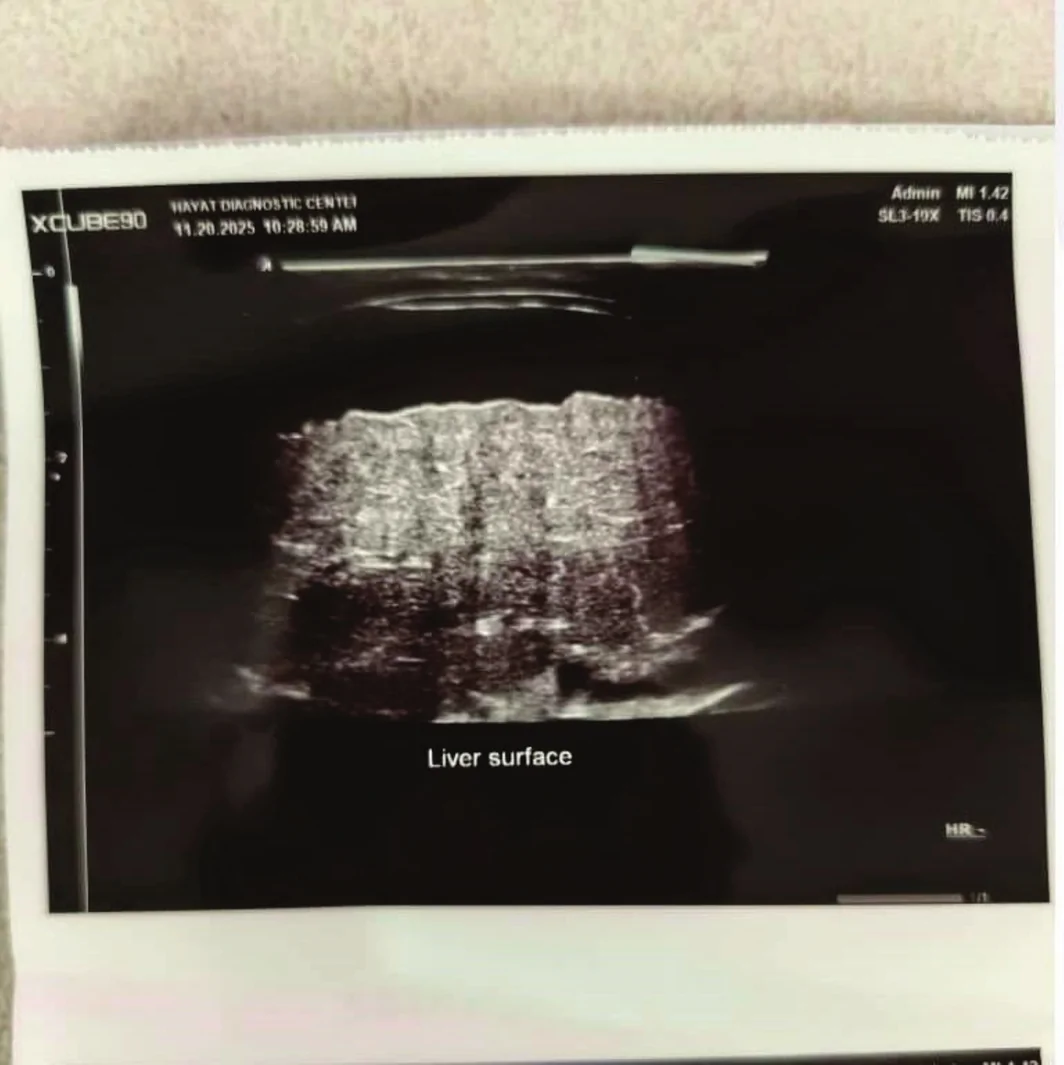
Grayscale abdominal ultrasonography demonstrating an irregular hepatic surface, consistent with chronic parenchymal liver disease, adjacent to anechoic free intraperitoneal fluid. The calibrated depth scale generated by the ultrasound system is displayed along the image margin and serves as the scale reference.
